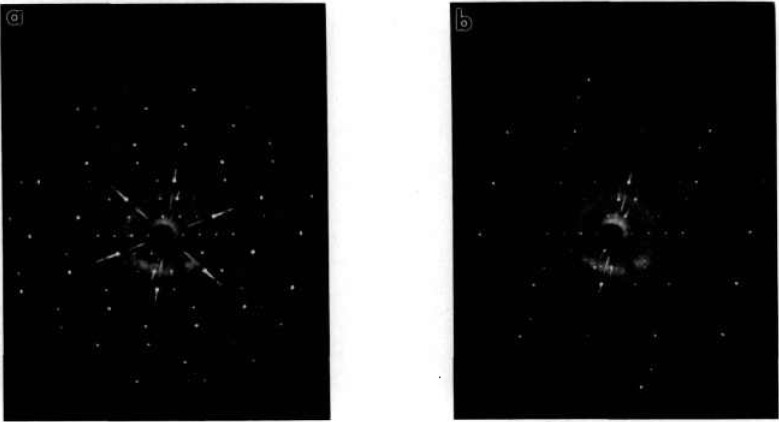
X-ray precession photographs of Ca_2_Bi_2_O_5_ (Mo radiation) (a) (*hk0*), (b) (*h0l*).
